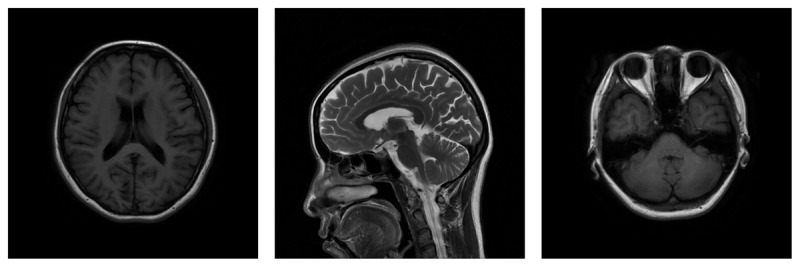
MRI shows no cerebral atrophy, no cerebellar atrophy, or other structural abnormalities.
